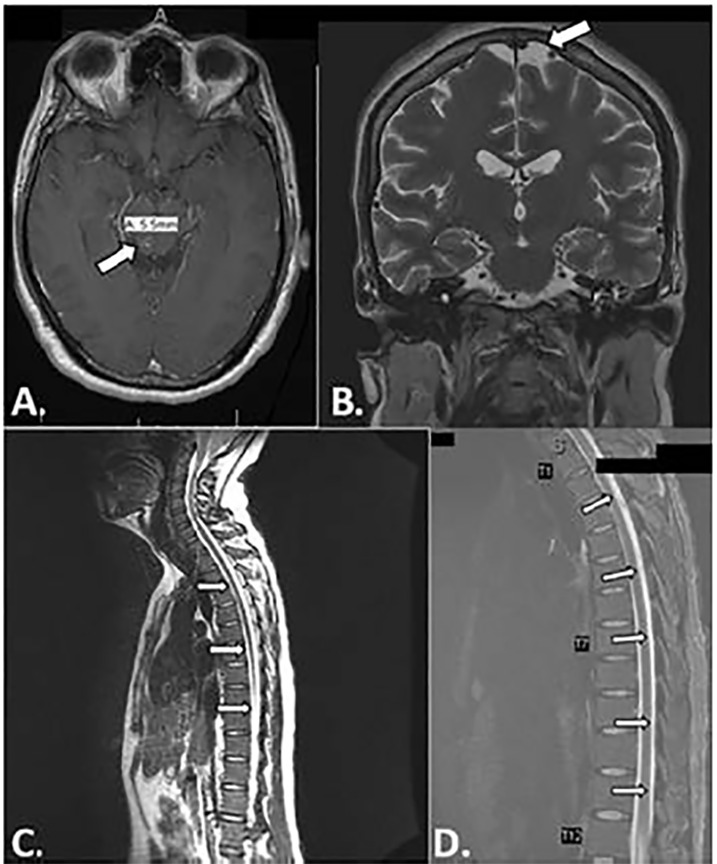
Magnetic resonance imaging of the brain, cervical spine and thoracic spine: A) axial view of the brain showing 5.5 millimeter (mm) small ring-shaped enhancement in the posterior right side of the midbrain potentially indicating infection; B) coronal view of the brain showing 7.4 mm incidental arachnoid cyst of the left frontal lobe; C) sagittal view of the cervical and thoracic spine showing smooth enhancement along the pleural surface of the thoracic spinal cord; D) magnified sagittal view of the thoracic spine showing enhancement along leptomeninges potentially indicating meningitis.
